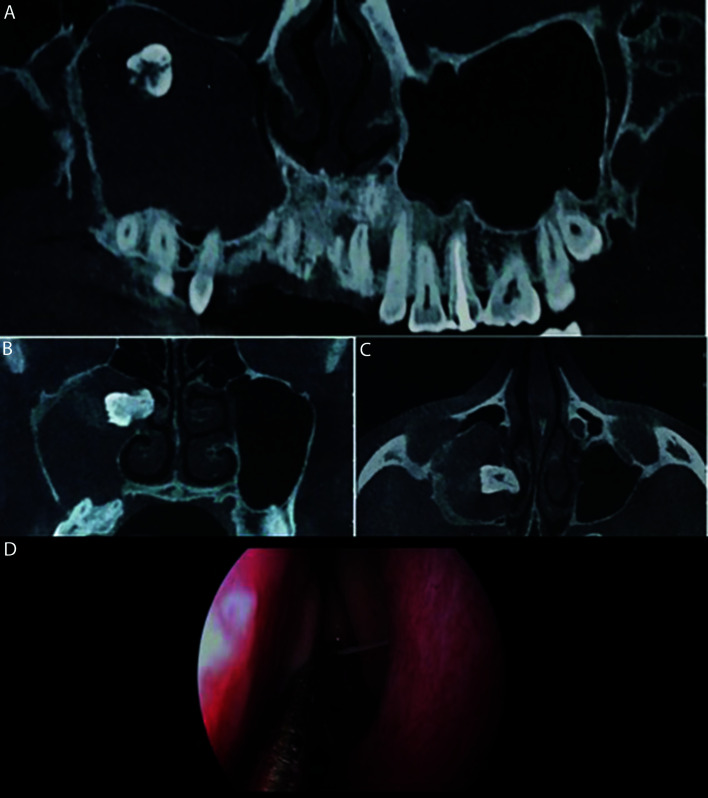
Recovery of the tooth through the nasal route (endoscopy). (A) CBCT panoramic reconstruction (B) Frontal CT scan (C) Axial CT scan. Note the tooth displaced and the signs of pathology in the right maxillary sinus. (D) Endoscopic approach view through the nasal route.
